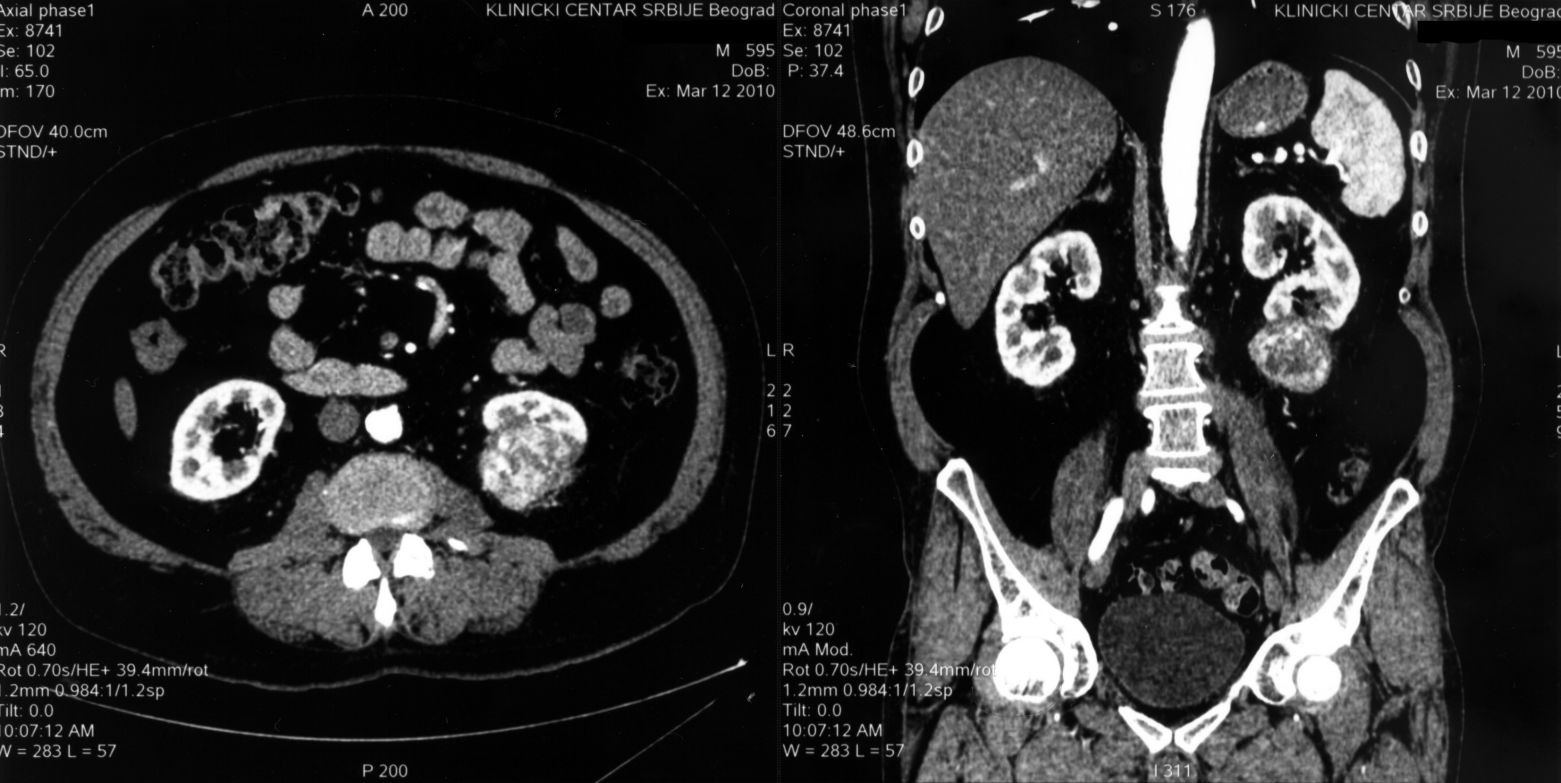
Abdominal CT scan revealing an infiltrating mass in lower portion of the left kidney.
